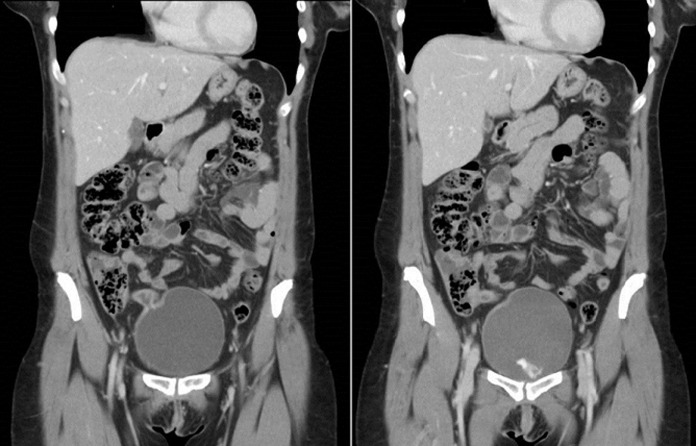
coronal multiplanar reconstruction (MPR) of the computed tomography images showing the fistula between the ileocecal junction and the bladder, pointed by arrows
